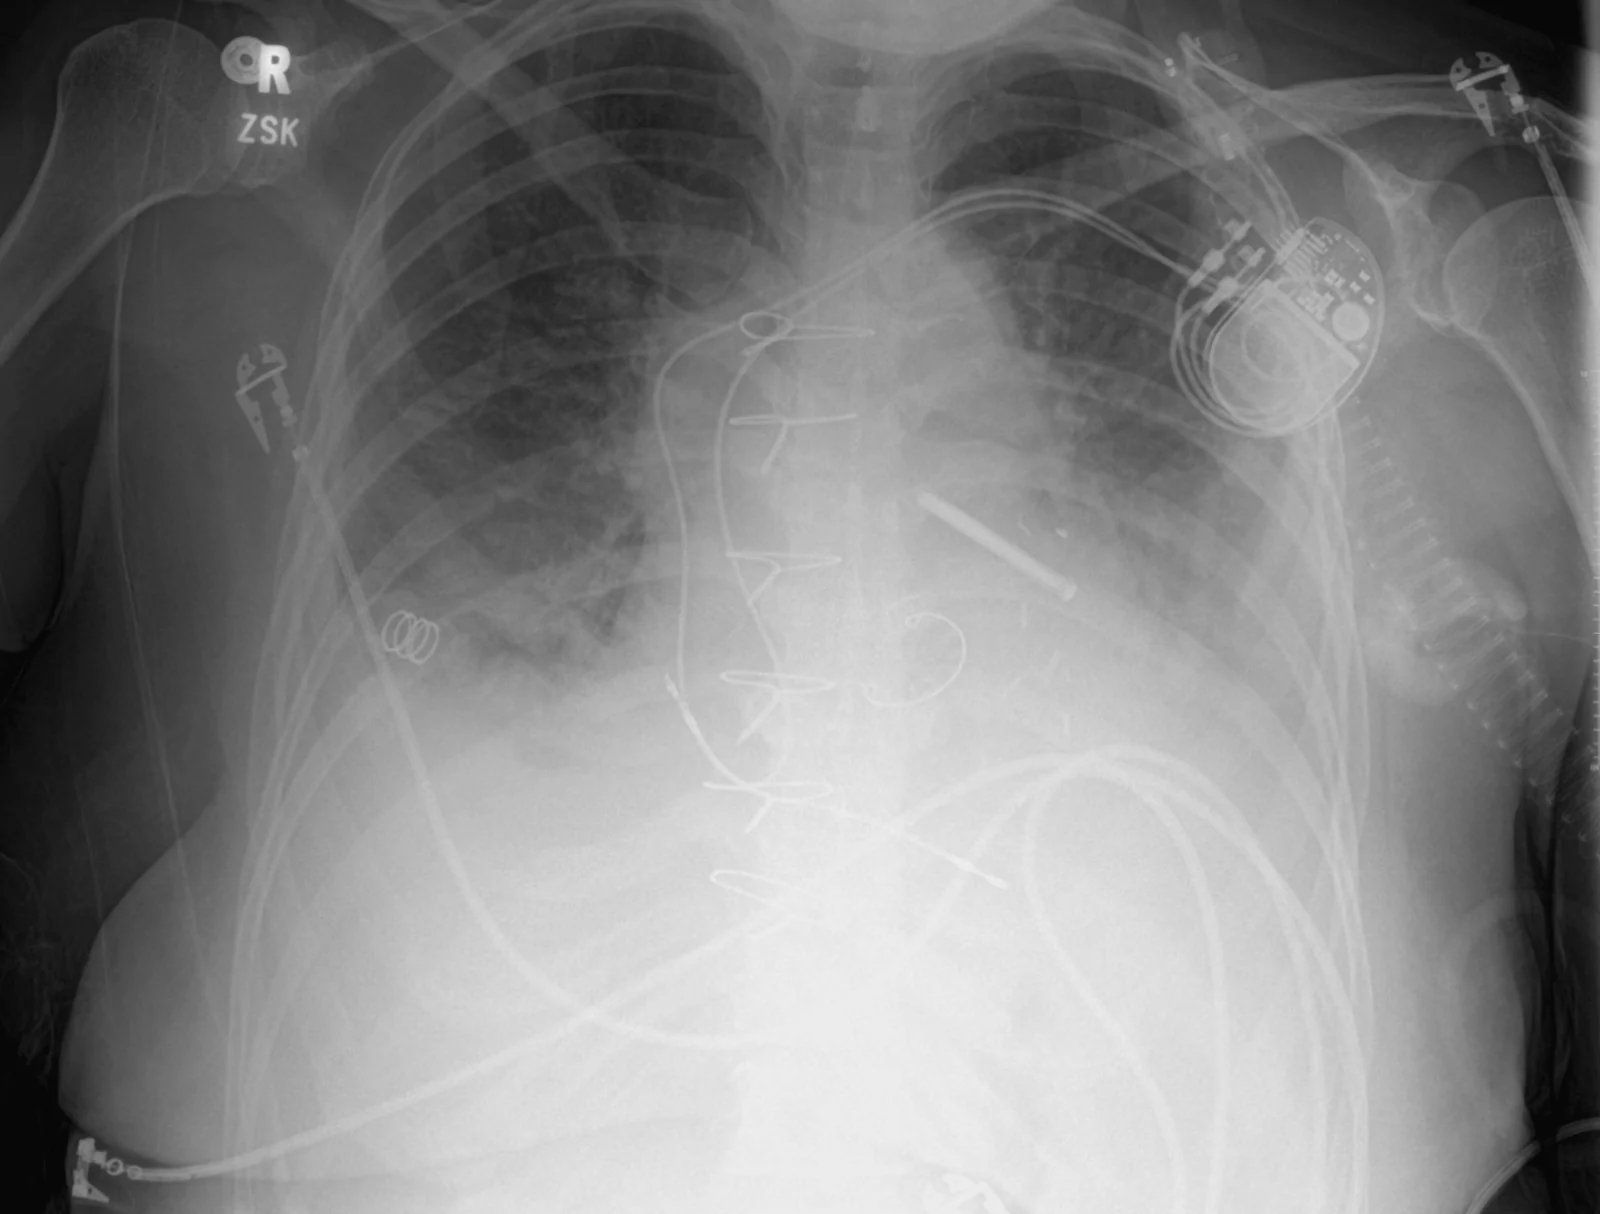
Follow-up chest radiograph 24 hours post-thoracentesis demonstrating interval improvement and stabilizing bilateral pulmonary infiltrates following ICU support and IV diuresis.
